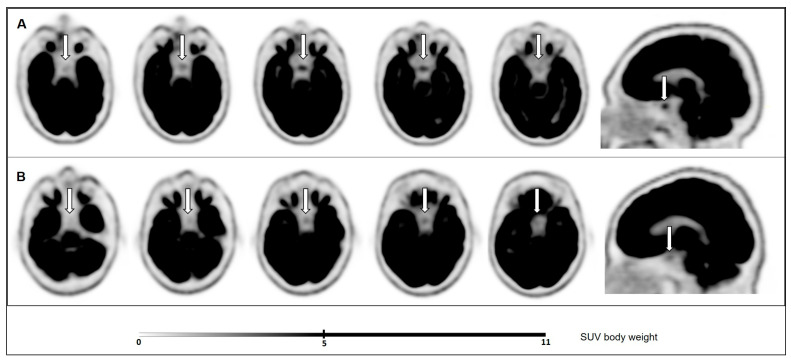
Pituitary gland (white arrows) ^18^F-FDG uptake signal in axial and sagittal planes in (A) a representative patient under THW (SUVmax = 4.4, SUVratio = 0.54); and (B) a representative patient under rhTSH stimulation (SUVmax = 3, SUVratio = 0.34).
